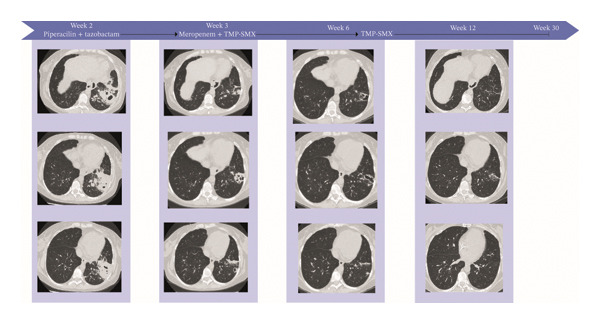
Timeline of the chest‐CT evolution and antimicrobial treatment. Weeks reflect time from initial presentation.
